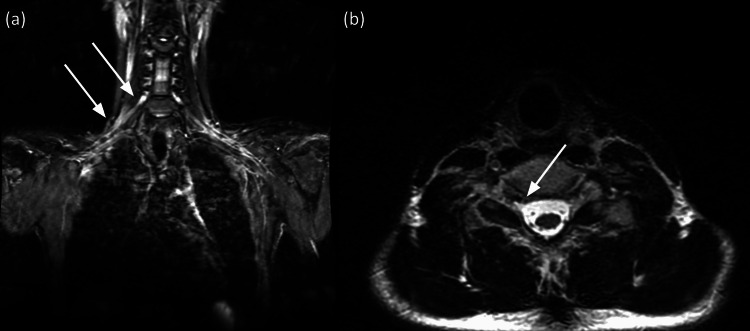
MRI of the brachial plexus and cervical spine (A) Coronal T2 STIR MRI of the brachial plexus. (B) Axial CUBE T2 MRI of the cervical spine. STIR: short tau inversion recovery; CUBE: coherent, ultrafast, beam, echoes; MRI: magnetic resonance imaging
